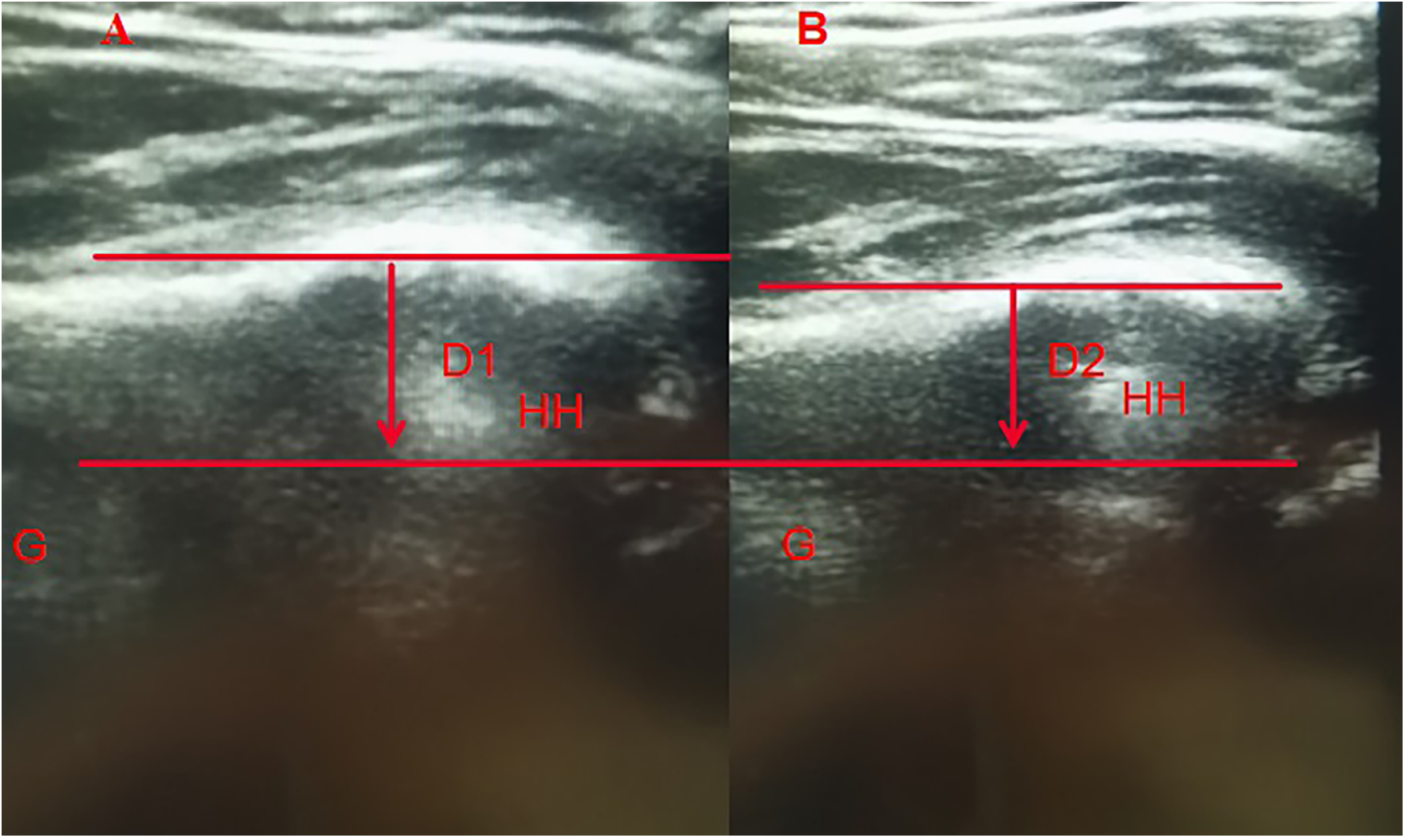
Ultrasonographic images figure (A) does not give a distraction and figure (B) gives a 40-N distraction. Two parallel lines were drawn through the posterior edges of glenoid and HH. The shortest distance between the 2 lines was measured both without (*D1*) and with (*D2*) 40-N distraction. Anterior HH translation was calculated by subtracting *D2* from *D1*. HH, humeral head; G, glenoid.
